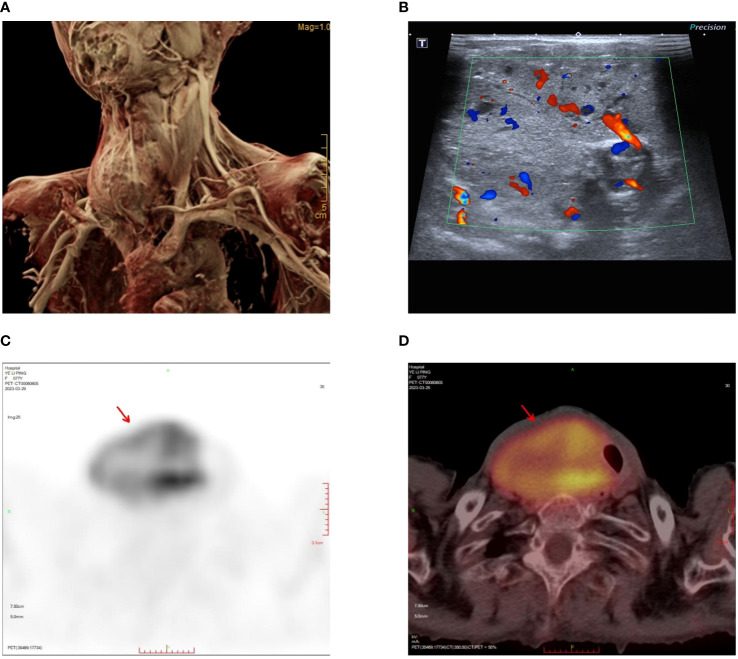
Tumor location and preoperative examinations. (A) MRI reconstruction showing the size and location of the tumor. (B) Neck ultrasound showing abundant blood supply to the tumor. (C) The maximum intensity projection (MIP) images summarizing the pathological distribution of [18F]-FDG in the tumor. (D) PET/CT scan showing heterogeneously intense FDG uptake in tumor.
